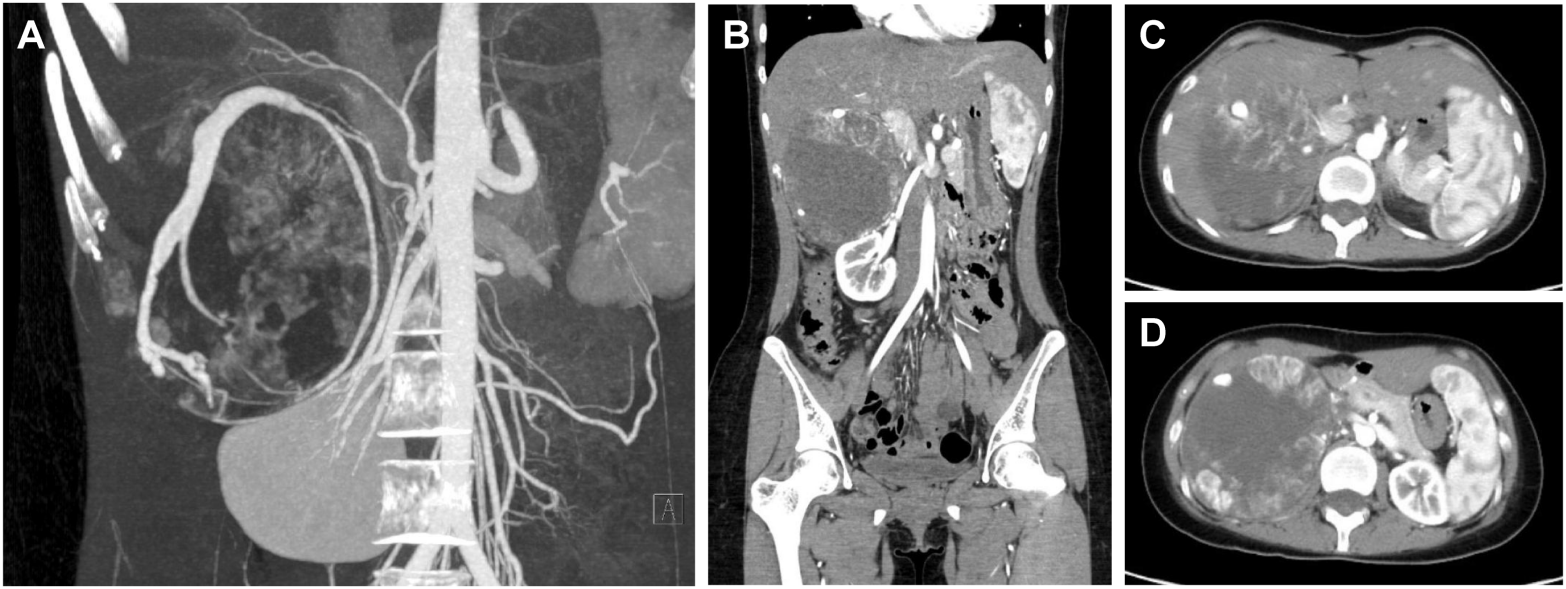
CT of a giant PCC in the right perirenal area. (A) CT of PCC angiography. Tortuous vessels with multiple aneurysmal-like dilatations were seen around the tumor. (B) Coronary position of the PCC. The tumor had a vague boundary with the liver and was close to the right renal pedicle. 10.9cm×9.0cm×10.7cm in size. (C) The upper part of the PCC was close to the inferior vena cava. (D) The maximum cross-section of the PCC was about 10.9cm×10.7cm.
